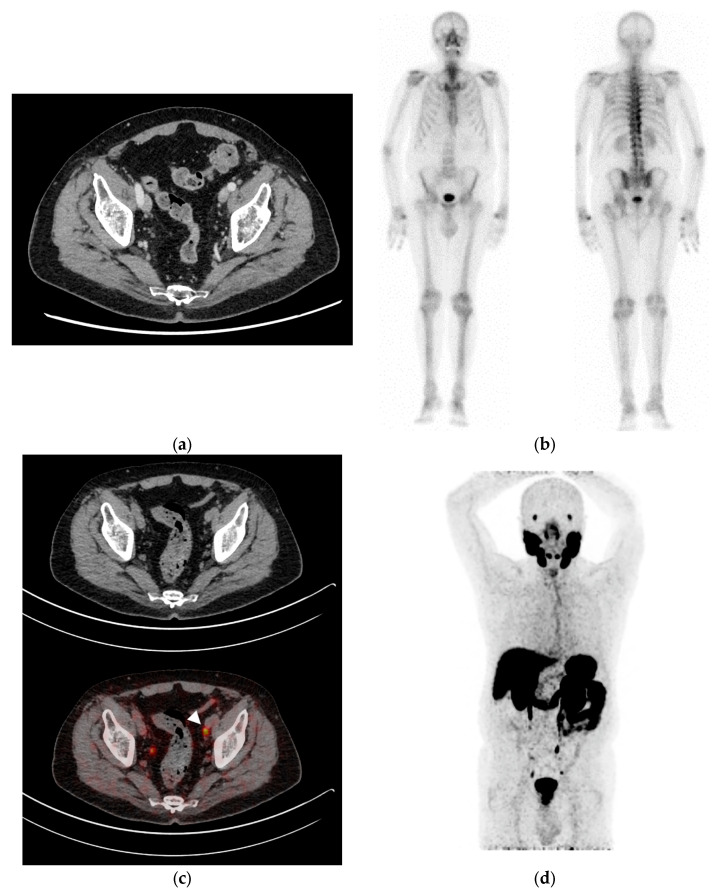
Clinical case: 82-year-old patient, iPSA 11.4 ng/mL, Gleason score 9 (5 + 4), with no pathologic findings at CT (a) and bone scintigraphy (b). (c,d) ^68^Ga-PSMA-11 PET/CT: left external iliac lymph node metastasis (5 mm) (arrowhead).
